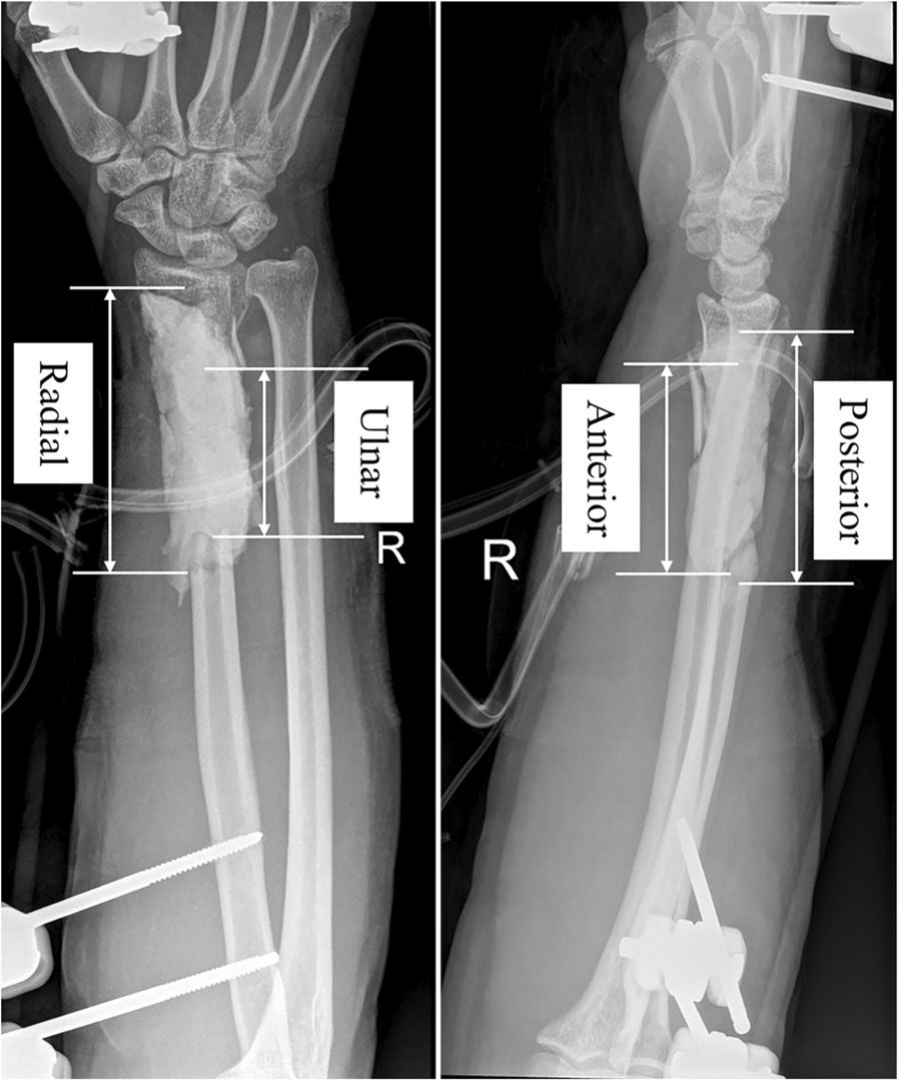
The size of the cortical gap was measured at the perimeter of the cortical bone on the radial, ulnar, anterior, and posterior cortical projections on anteroposterior and lateral radiographs, based on the method reported by Haines et al. [14]. These measurements were averaged over four cortices to obtain the radiographic apparent bone gap for each fracture
